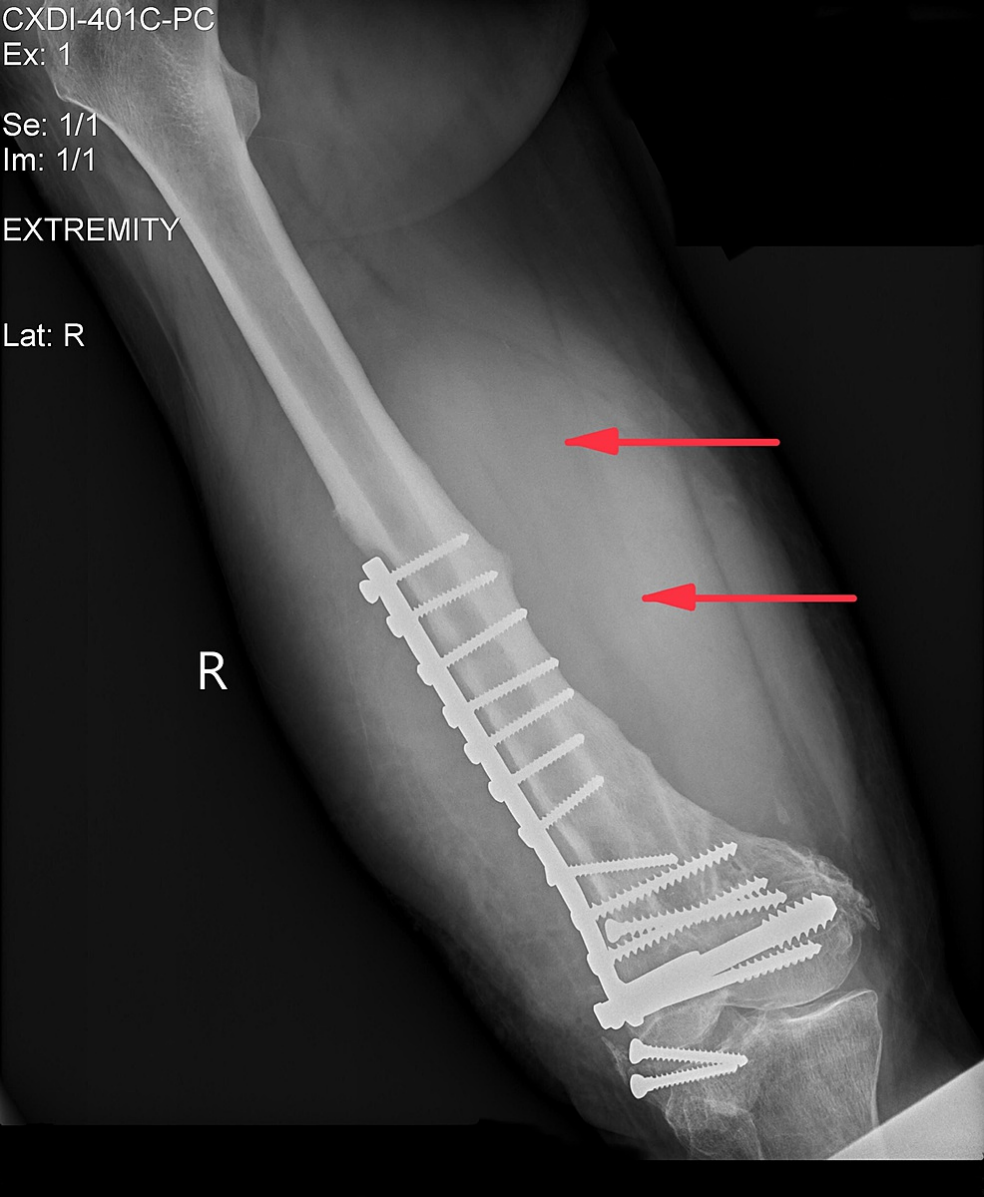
X-ray of the right femur (AP view) showing DCP and surrounding hyperlucency. AP - anteroposterior,  DCP - dynamic compression plate
